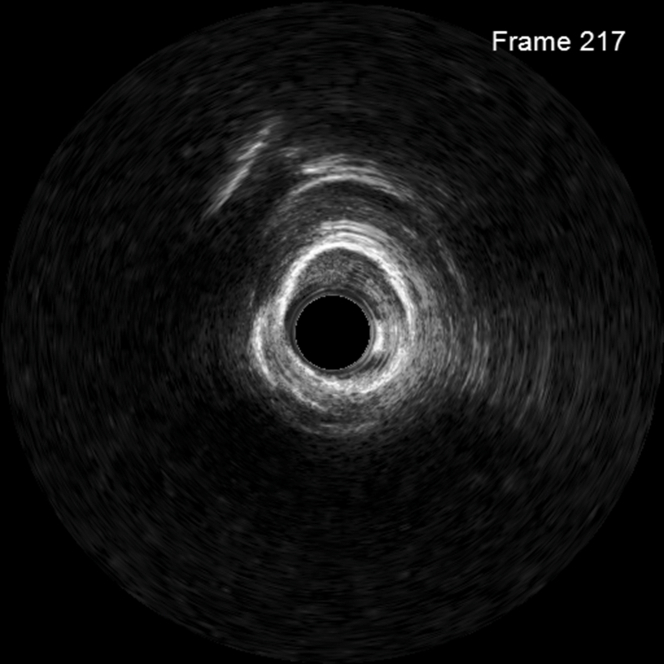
Intravascular Ultrasound Demonstrating No Calcium Fracture
